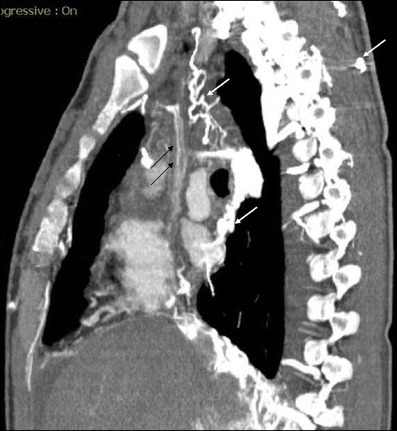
A 20-year-old male with Behçet's disease. Sagittal reformatted CT image shows superior vena cava thrombosis (*black arrows*) and collateral vessels in the mediastinum and chest wall (*white arrows*)
